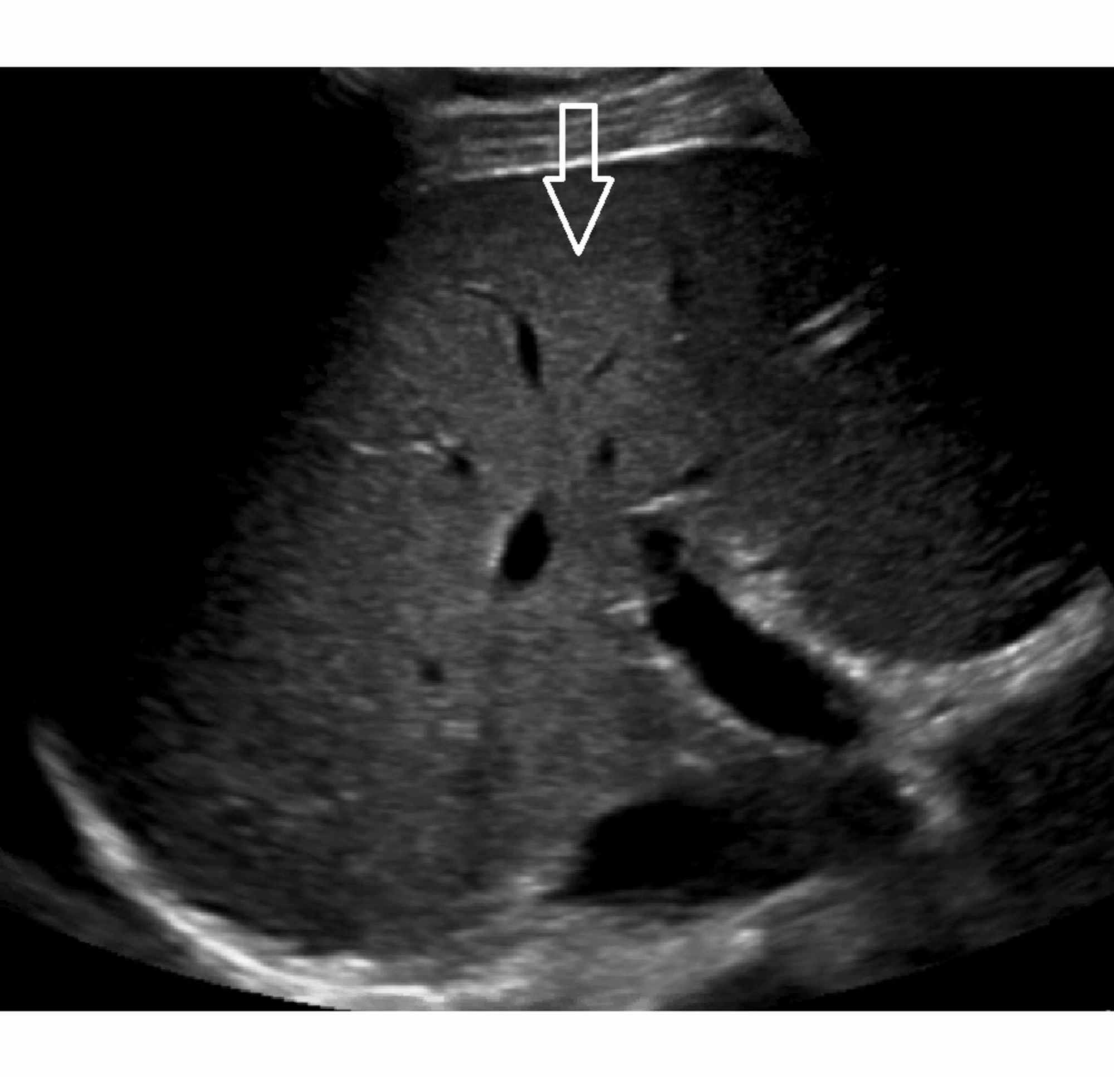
Right upper quadrant ultrasound showing grossly normal liver echo texture (white arrow demonstrating liver).
